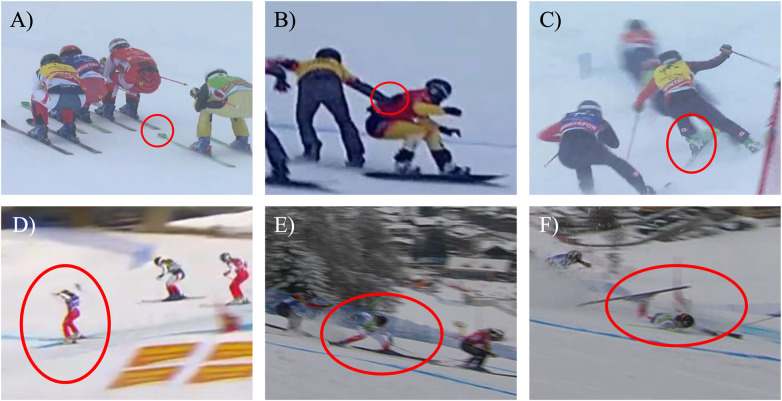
Examples for (A) RS, (B) CT, (C) ACT shown in upper row, from left to right and (D) OOB, (E) TNR, (F) CR, shown in lower row, from left to right.
